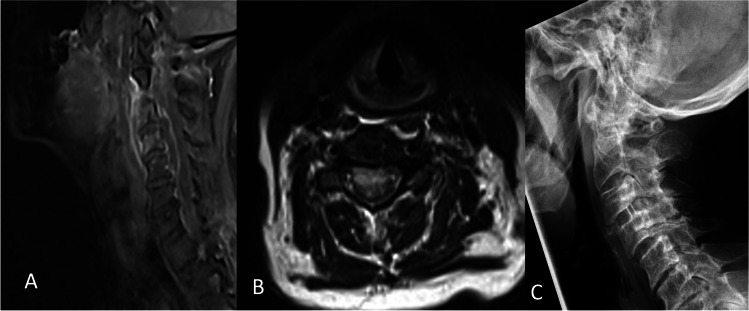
Postcontrast sagittal (A) and axial (B) T2-weighted magnetic imaging of ventral cervical epidural abscess of C3 and C4 of an 85-year-old male patient presenting with progressive tetraparesis. Lateral radiographic view of ACDF extending from level C3 to C4 with interbody graft seen at C3–C4 (C)
